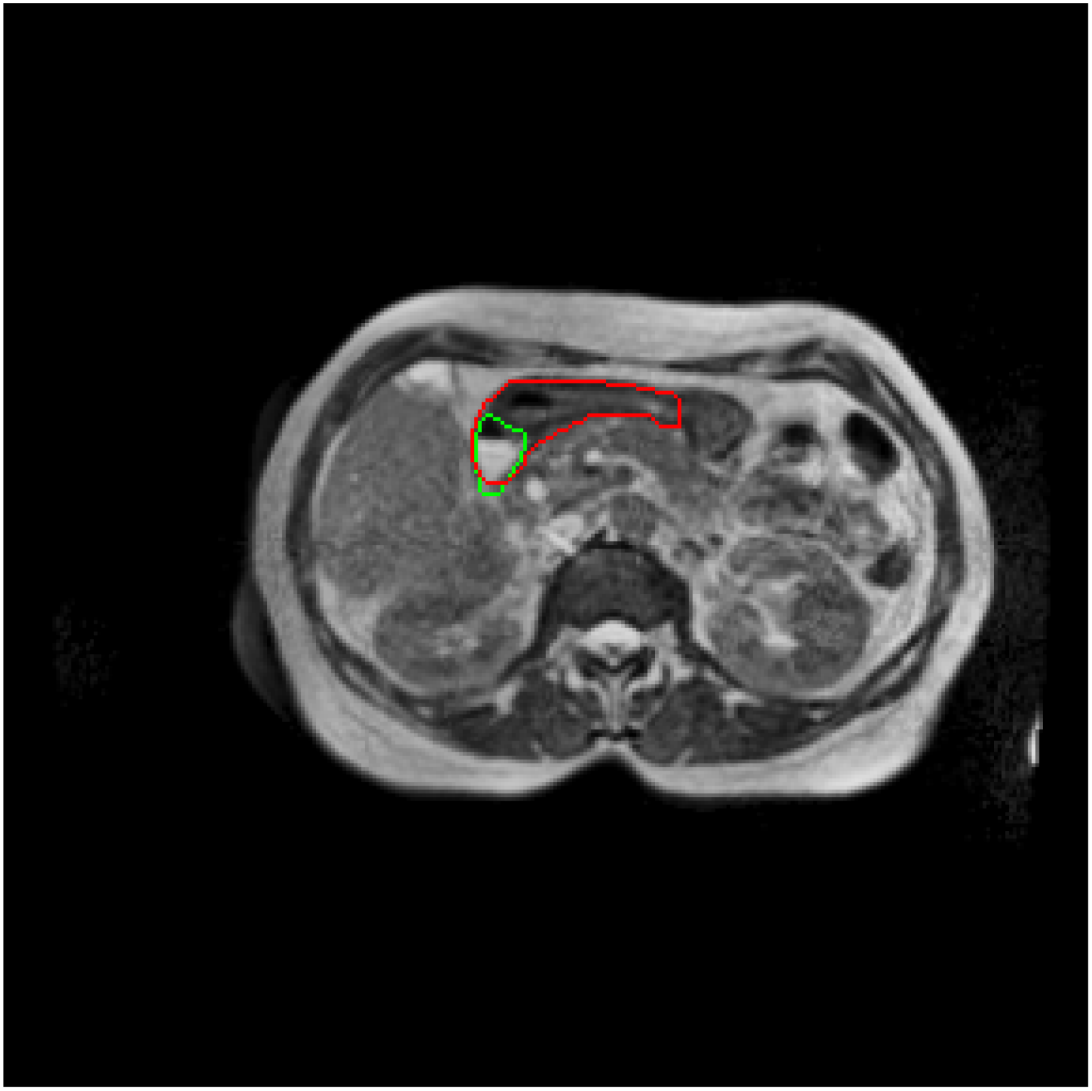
Manual segmentation of the duodenum. Segmentation of the same MR images by two different radiation oncologists.
